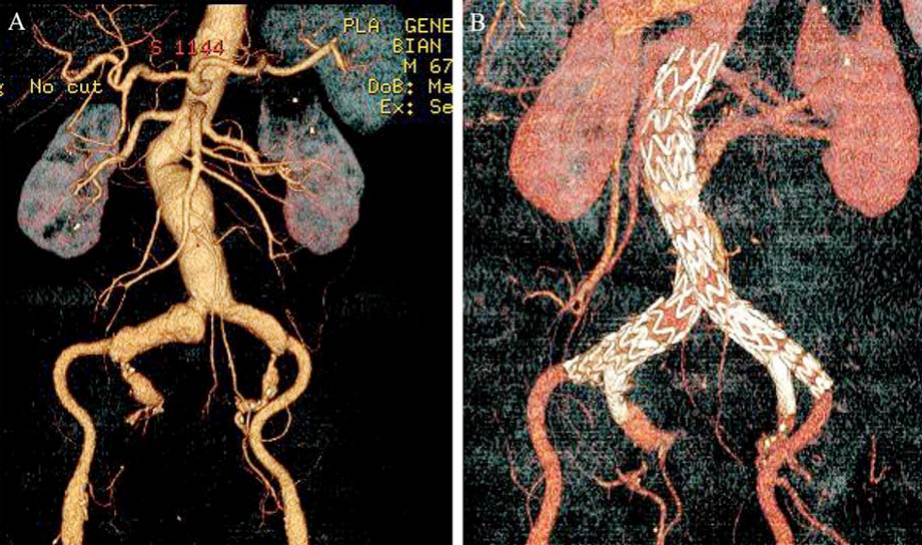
CT images for one patient who received EVAR. A: Preoperative CT discloses AAA complicated with bilateral iliac aneurysms; B: Re-examination in the postoperative 6th month shows well-maintained stent form without endoleak, and unobstructed bilateral internal iliac arteries.
